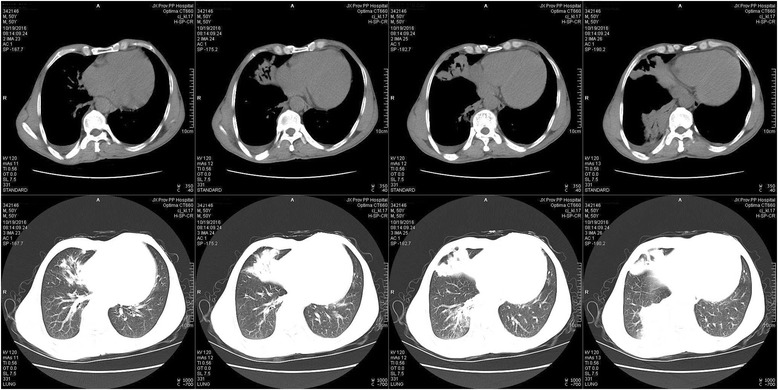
The second Chest CT (Oct. 19) showed the shadow had absorbed
